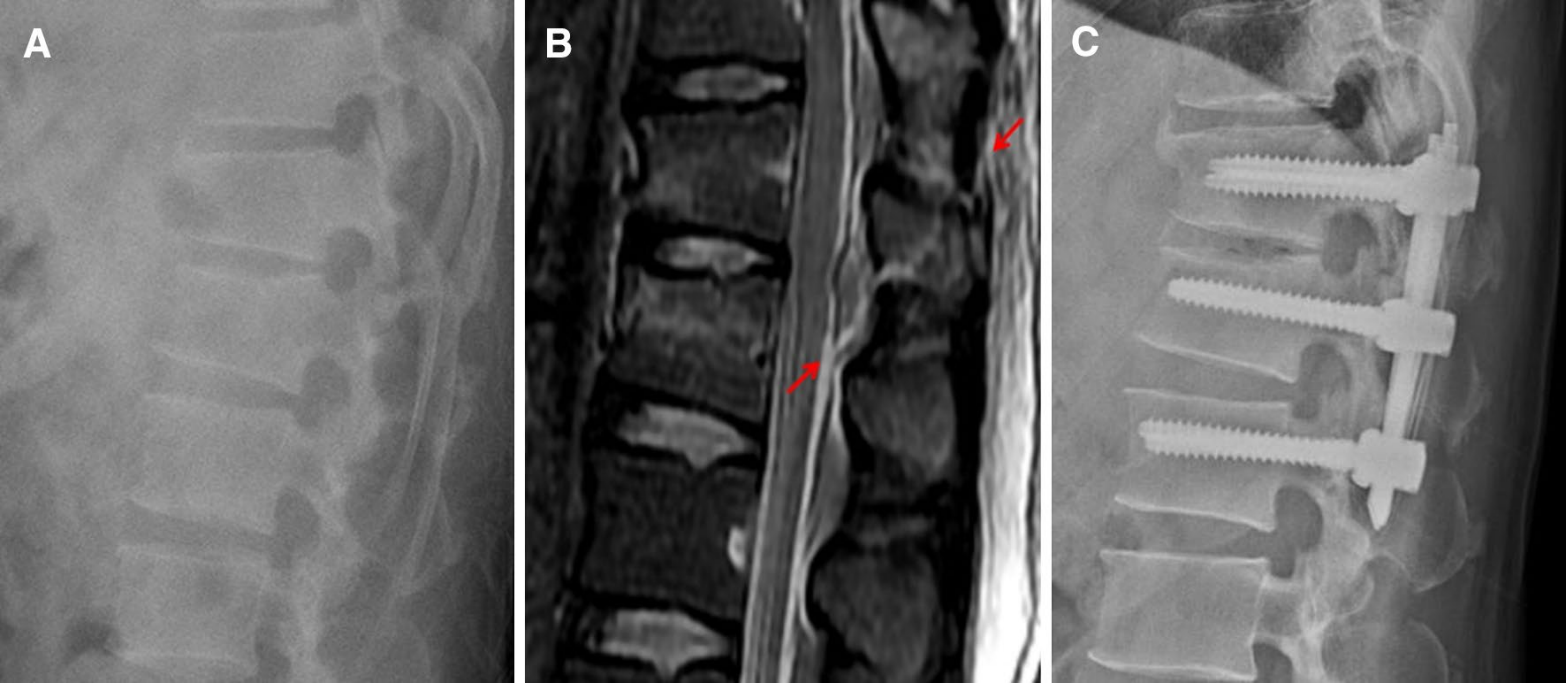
(A) A 46-year-old male patient underwent conservative treatment initially because the diagnosis was considered as L1 stable burst fracture on plain radiography. (B) The patient complained of more severe pain after 2 days and MRI was performed. MRI showed occult injury of posterior ligamentous complex (red arrow). (C) The patient underwent posterior fixation and plain radiography at postoperative 29 months showed well maintained vertebral height.
